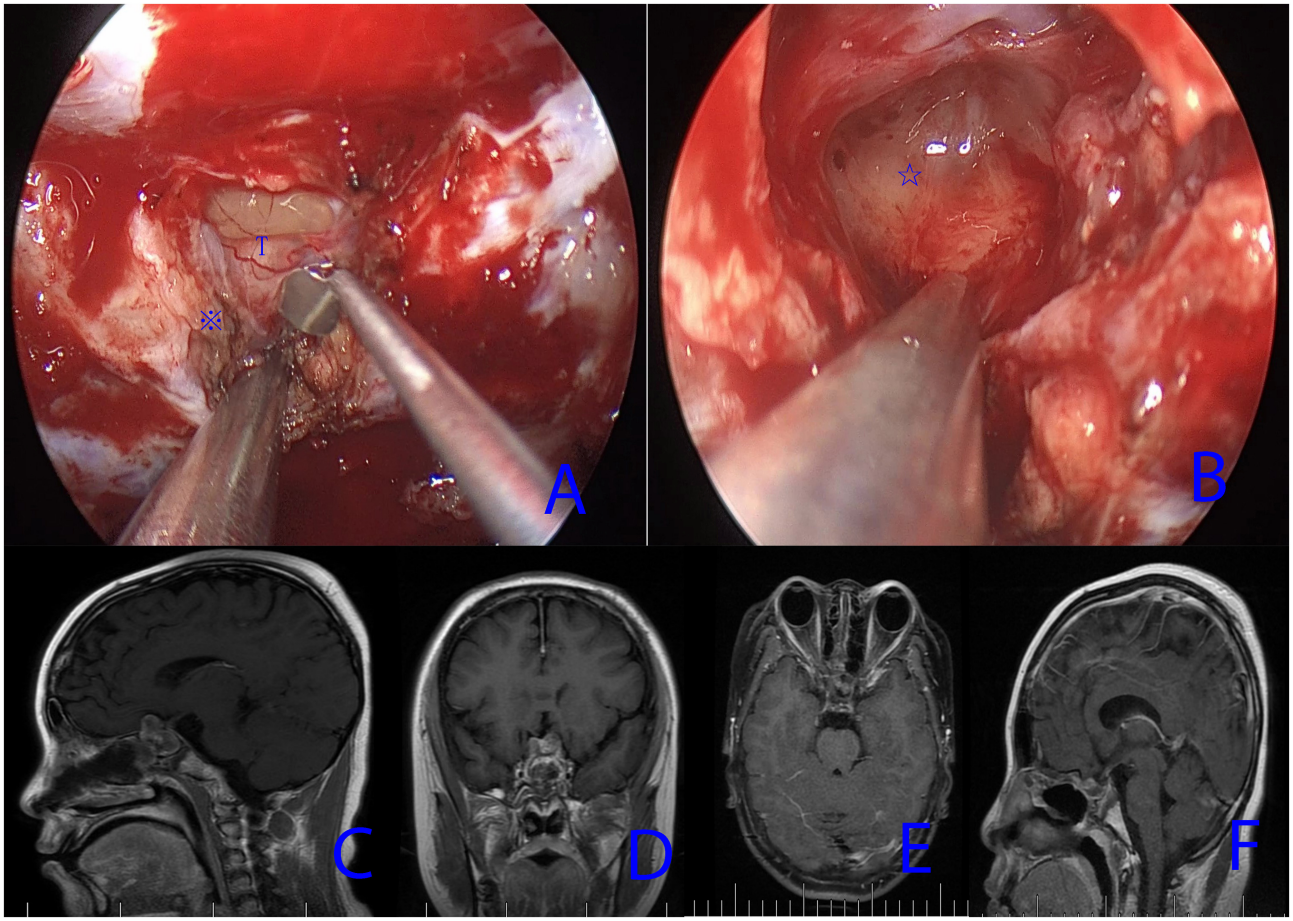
Case 11. (A,B) Intraoperative endoscopic views; Asterisk (※) indicates the scar fused with the dura; ⋆ Indicates the thickened arachnoid. T, tumor. (C,D) Preoperative MRI images (sagittal, coronal) showing recurrent intrasellar and suprasellar craniopharyngiomas. (E,F) Postoperative MRI images (axial, sagittal) demonstrating tumor removal.
